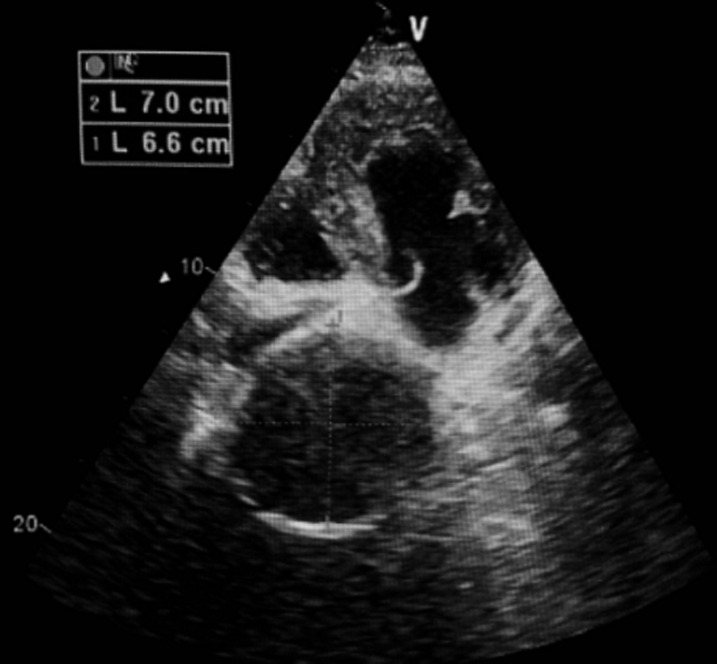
echocardiography: modified apical view showing an echolucent well defined space (70 x 66 mm) next both right and left atrium
